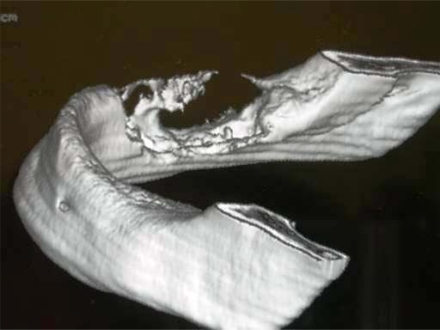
3D CT reconstruction of the internal (lingual) aspect of the jaw demonstrates massive destruction of the lingual cortical bone; vestibular cortical bone appears as an interrupted thin line. The three-dimensional picture points out loosening of the trabecular features of the jaw bone.
